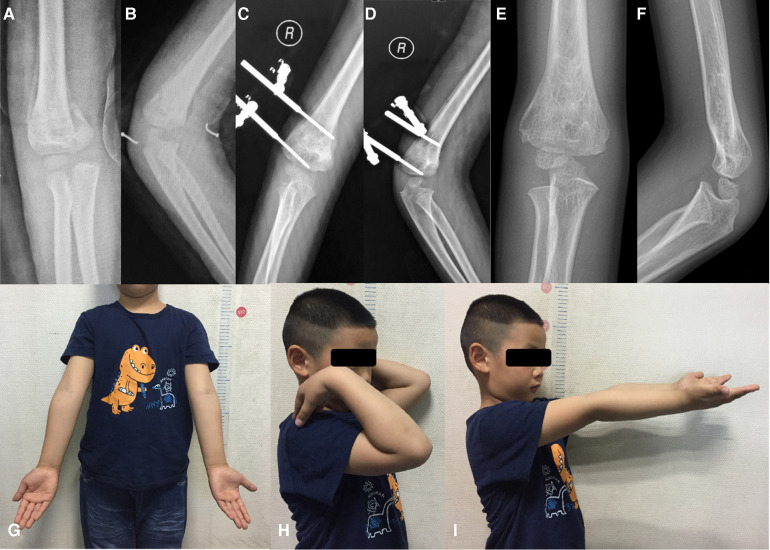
(A) anteroposterior and (B) lateral radiographs of the 5-year-old boy with delayed supracondylar fracture of right humerus; (C) anteroposterior and (D) lateral radiographs at 6 weeks post-operation; (E) anteroposterior and (F) lateral radiographs at 12 months post-operation; and the follow-up in 24 months after surgery show excellent cosmetic results (G) and the functional appearance (H,I).
